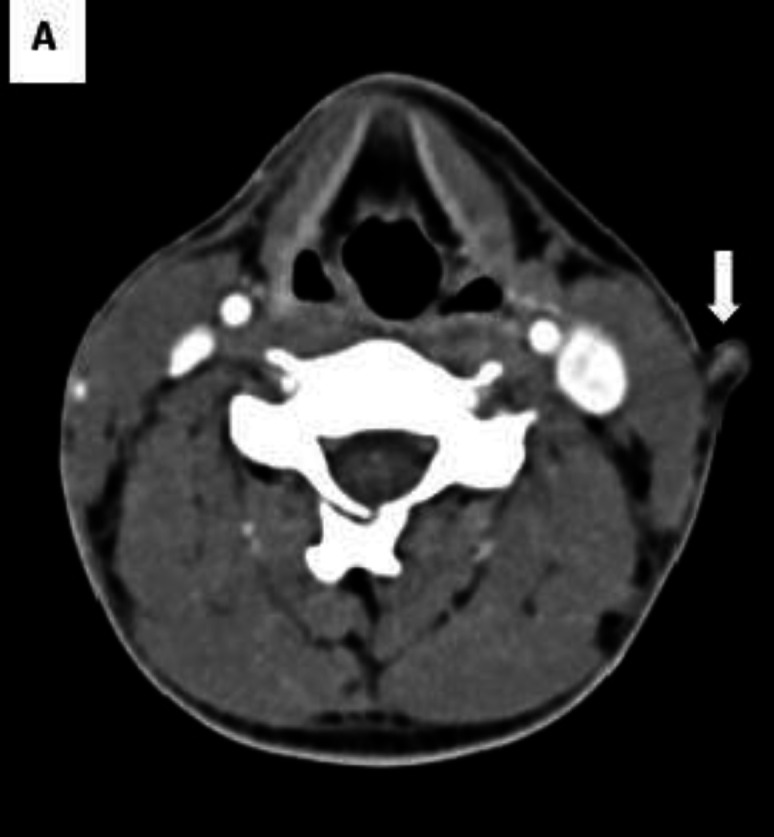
Spiral neck CT scan, axial view. The lesion with central cartilaginous density has been shown with an arrow
